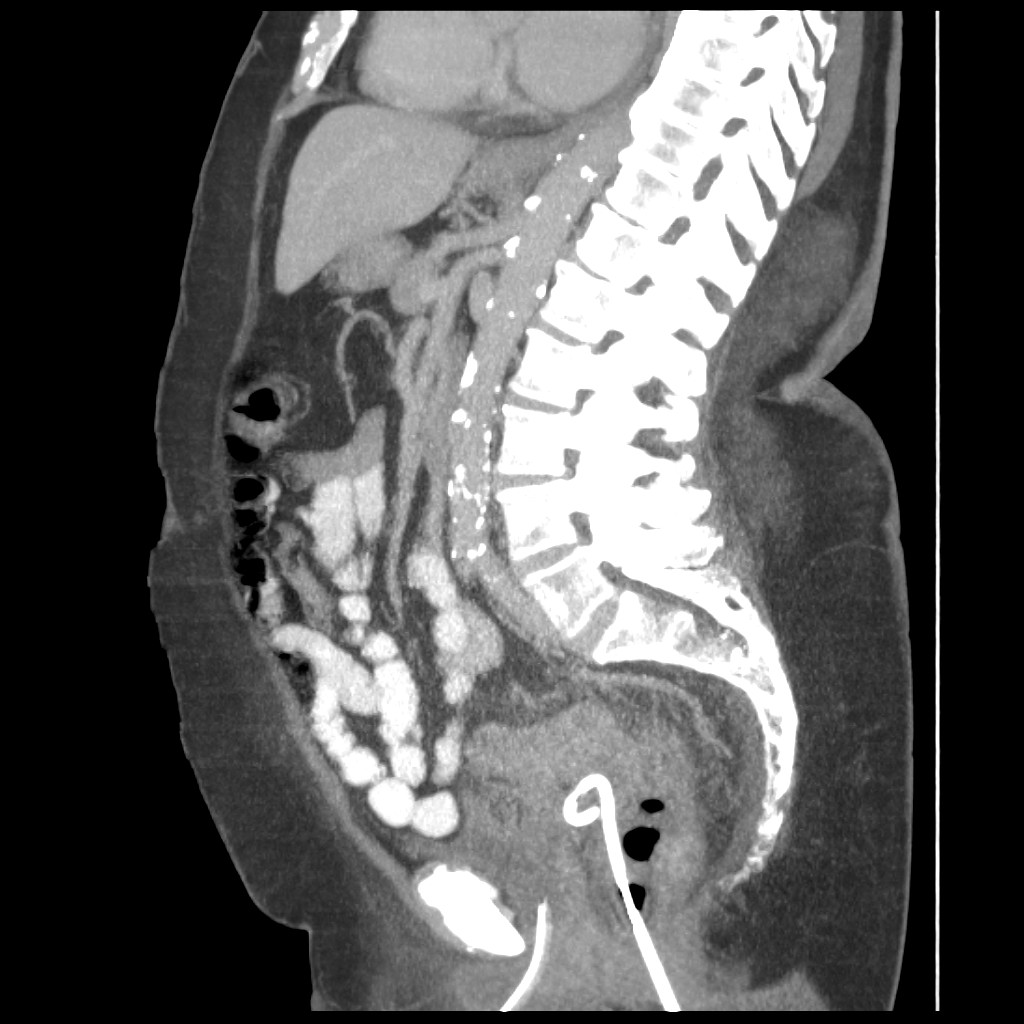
Computed tomography scan of the abdomen and pelvis (sagital section) demonstrating the transrectal placement of a pigtail catheter.
